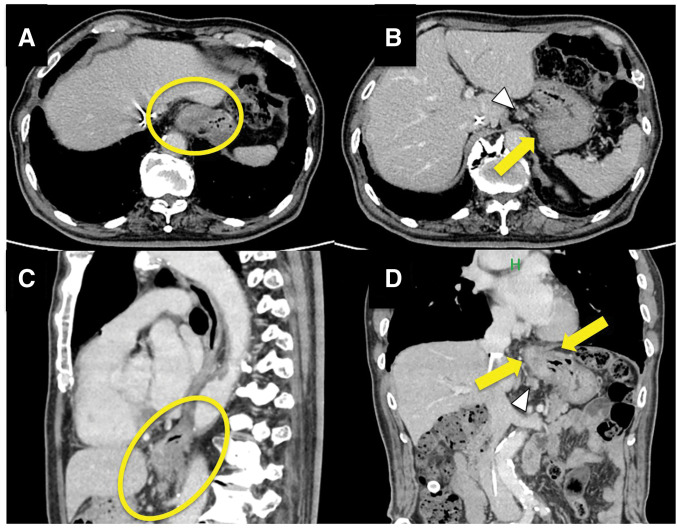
Contrast-enhanced CT of the chest and abdomen. The yellow circles in panels A and C indicate the primary tumor, and the yellow arrows in panels B and D indicate the enlarged perigastric lymph nodes. (A, B) Axial images reveal concentric wall thickening of the lower thoracic esophagus (A) and an irregular enhancing mass at the gastric cardia (B). The enlarged perigastric lymph node is indicated by an arrowhead in panel (B). (C) Sagittal reconstruction shows longitudinal tumor extension from the distal esophagus through the EGJ. (D) Coronal reconstruction demonstrates continuous tumor infiltration across the EGJ and enlarged perigastric lymph nodes (arrowheads), suggestive of regional nodal metastasis. EGJ, esophagogastric junction
